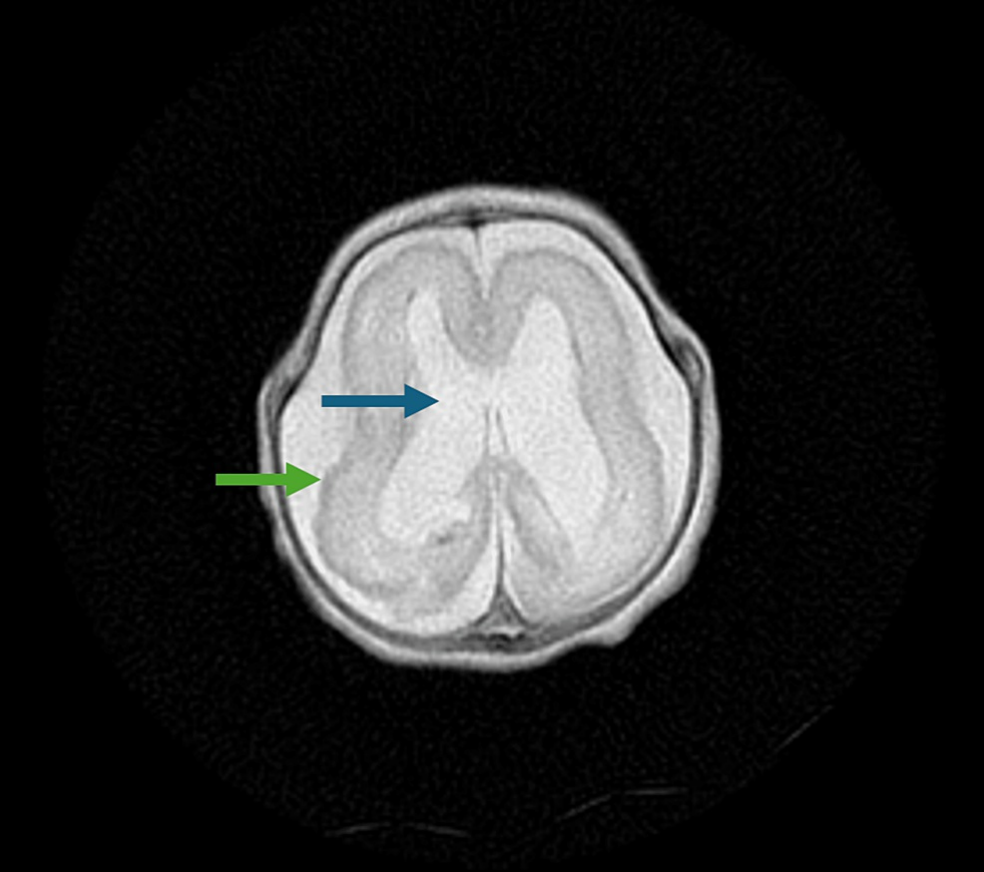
Axial plane of brain MRI at the day of life 31, displaying progression to lissencephaly (green arrow) and Grade 2 intraventricular hemorrhage with occipital horn-dependent blood (blue arrow)
